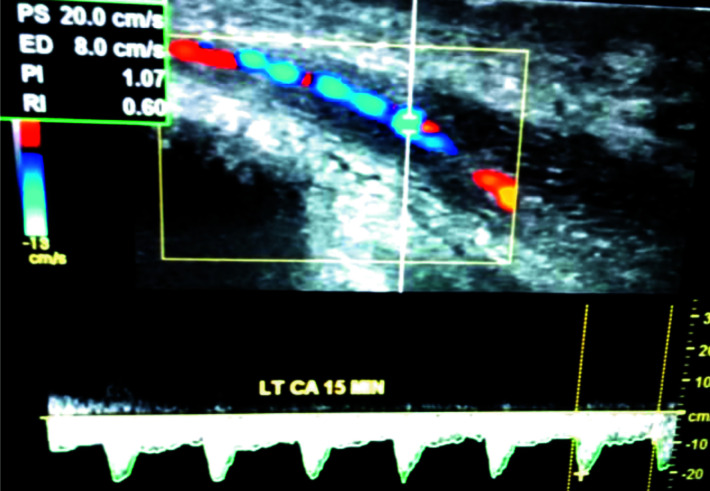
Left CA triplex Doppler waveform at 15 minutes showing multiple areas of narrowing and dilatations, giving a beaded appearance. Note the peak systolic velocity of 20cm/s, and end diastolic velocity of 8cm/s
